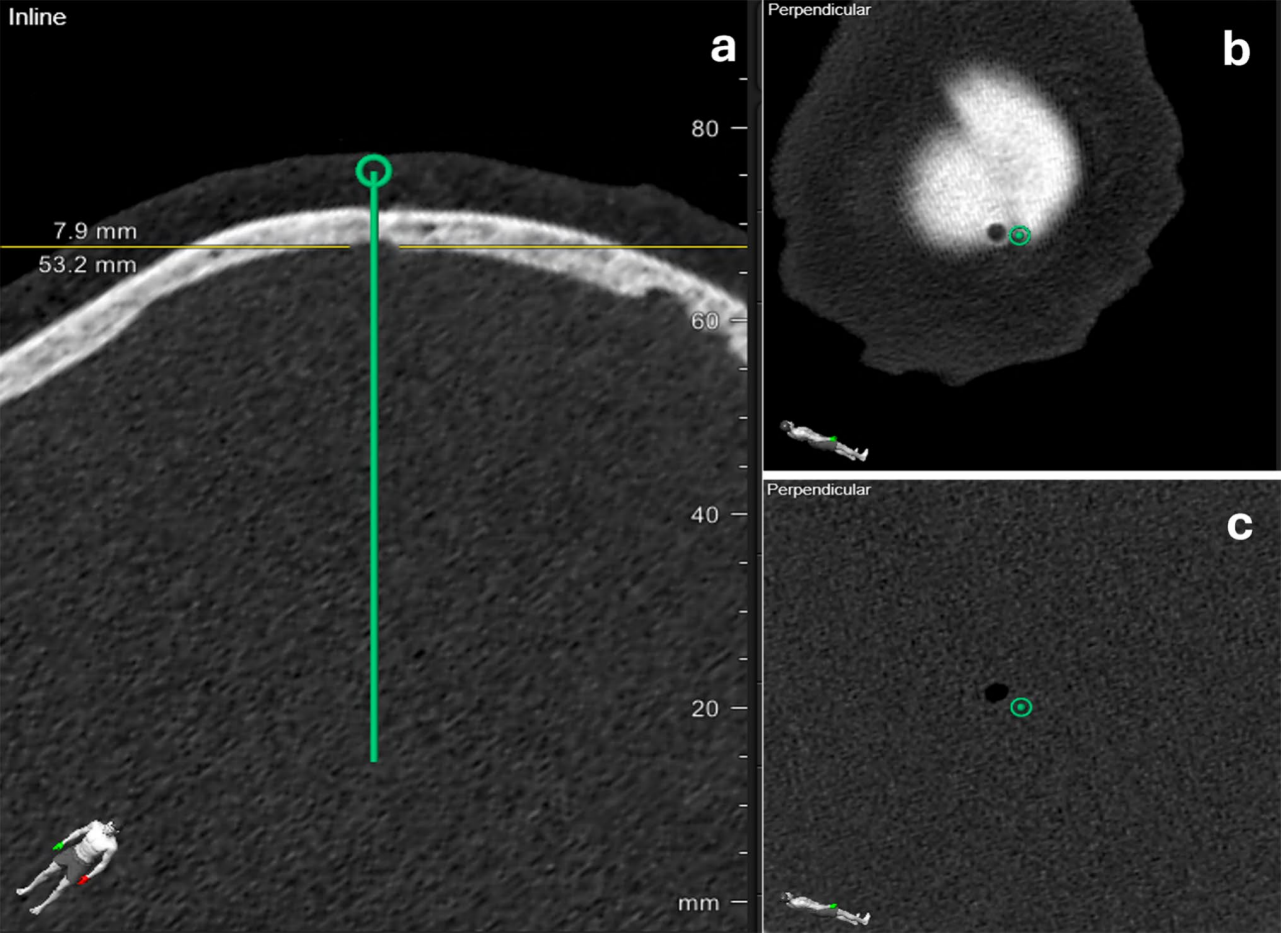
Postoperative CT scan demonstrating the inline (a) and perpendicular (b, c) views of the planned biopsy trajectory. In the linear view (a), the distance from the internal table of the skull to the planned target point was measured to assess target depth (53.2 mm). In the orthogonal views (b, c), deviations between the planned entry point and the actual burr hole, as well as between the planned target point and the actual biopsy cavity (air bubble), were evaluated to determine stereotactic accuracy
